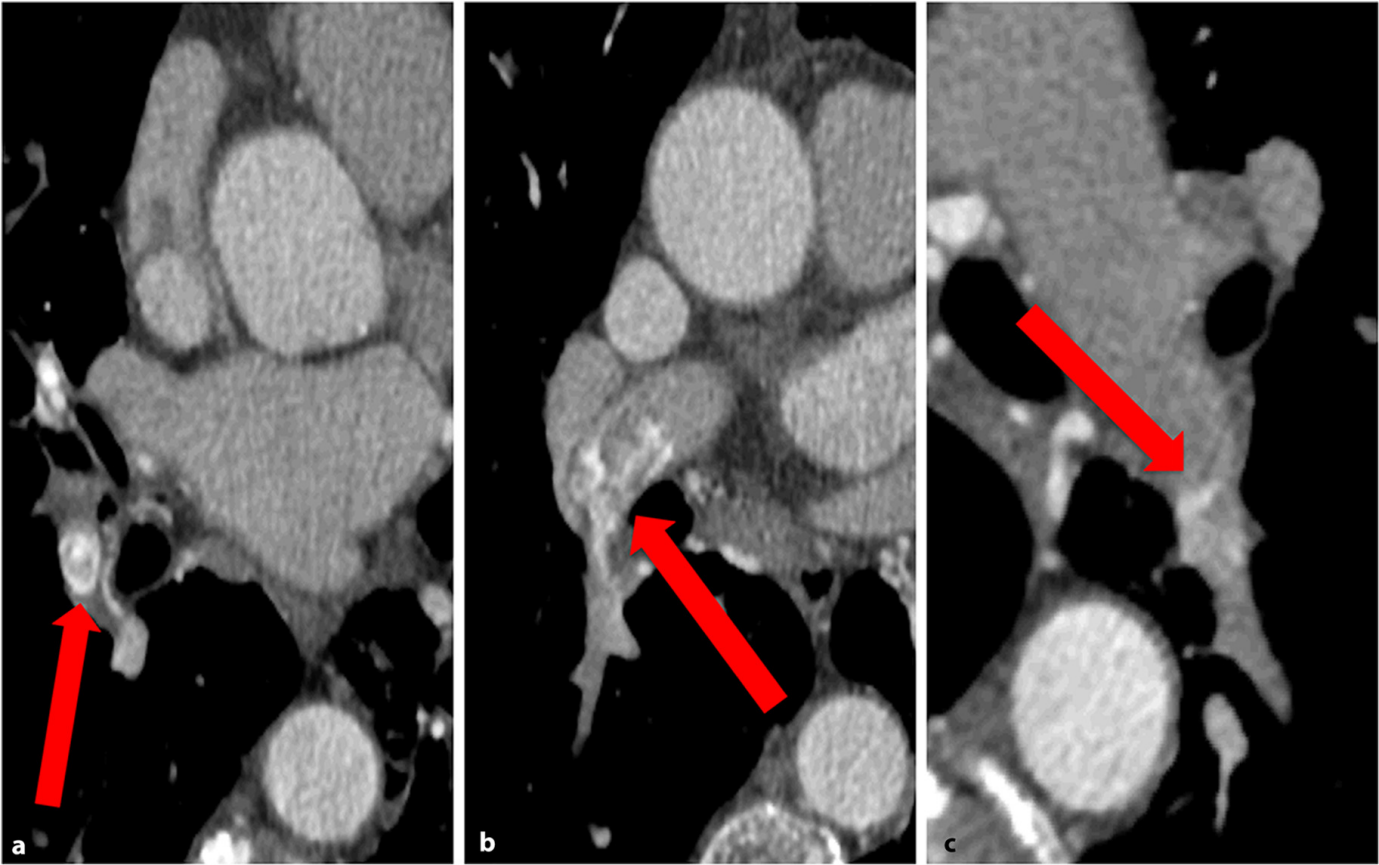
(a–c) Axial slices from the CT aortic angiogram showing reversal of the initial findings with systemic arterial contrast forming a positive filling defect against the relative negative contrast in the pulmonary arteries (red arrows).
